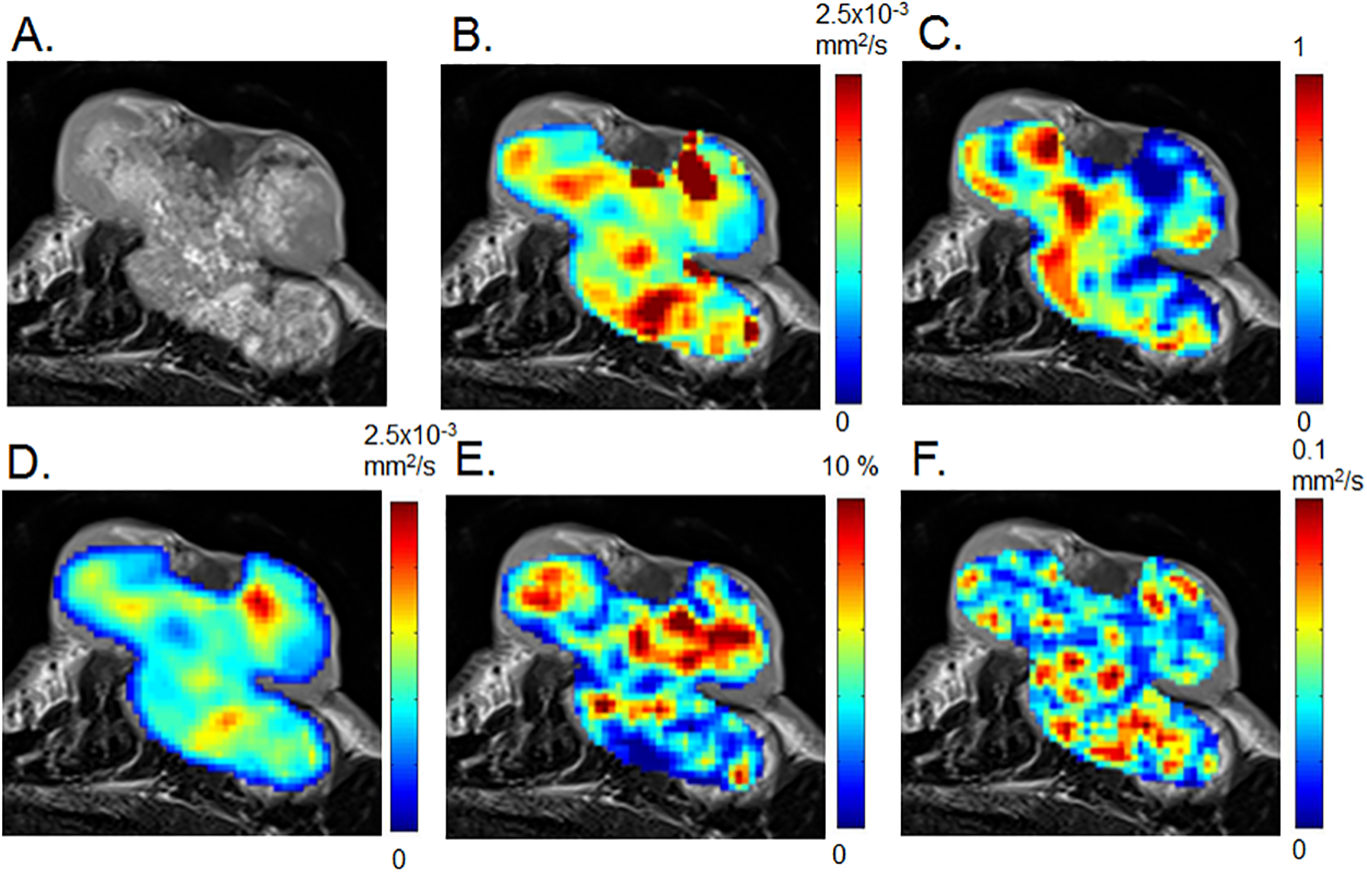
DWI and IVIM parametric maps of invasive ductal carcinoma in a 63-year-old woman. The patient had bilateral lesions involving invasive ductal carcinoma in the left breast and fibroadenoma in the right breast (Fig 1). Axial diffusion and IVIM MRI maps were overlaid on T2-weighted images. (A) T2-weighted image, (B) ADC_0_ map, (C) K map, (D) sADC map (E) fIVIM map, (F) D* map.
